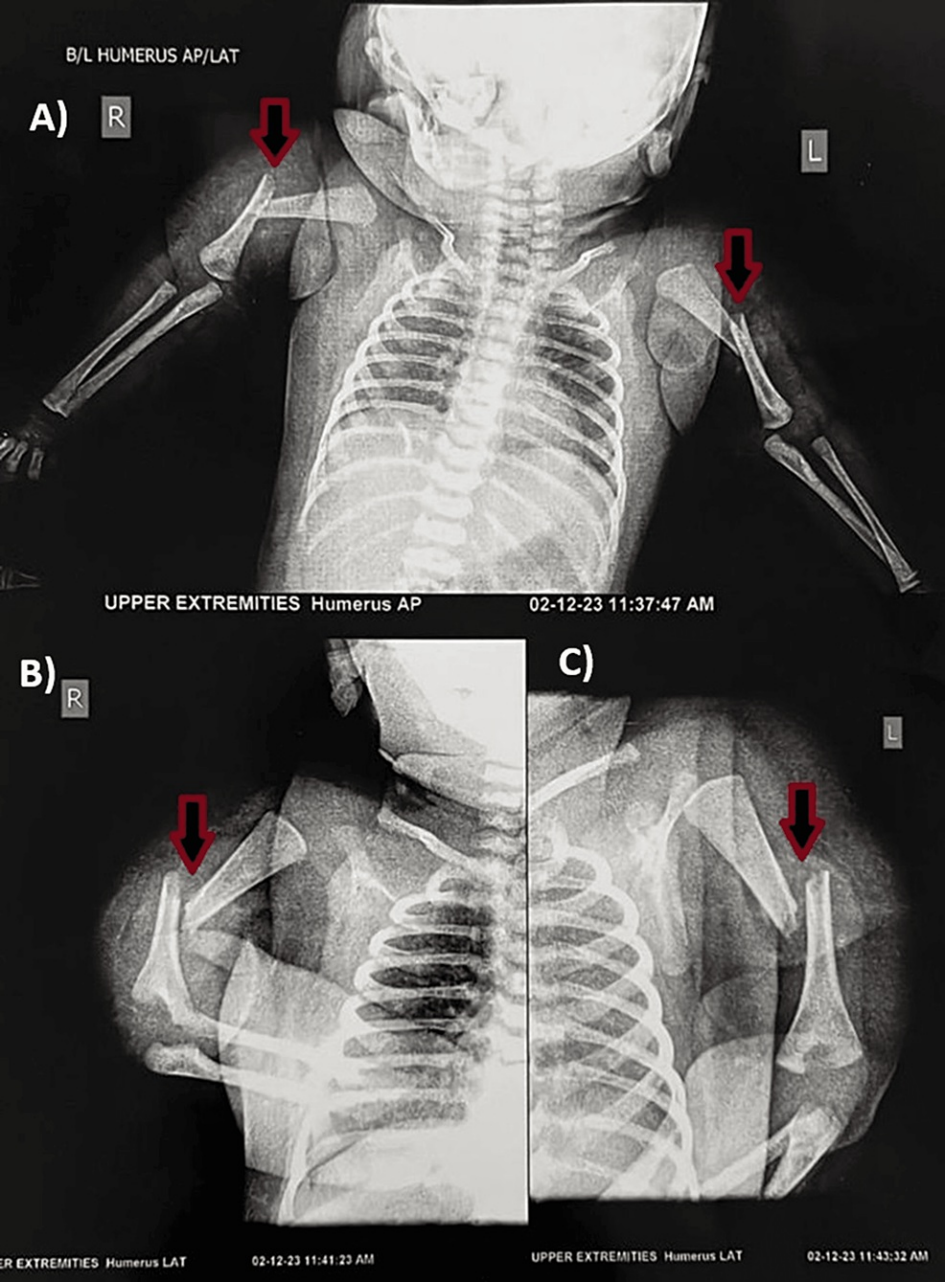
X-ray confirming bilateral humerus midshaft fracture A) Bilateral humerus midshaft fracture anterior-posterior (AP) view; B) right humerus midshaft fracture lateral view; C) left humerus midshaft fracture lateral view
